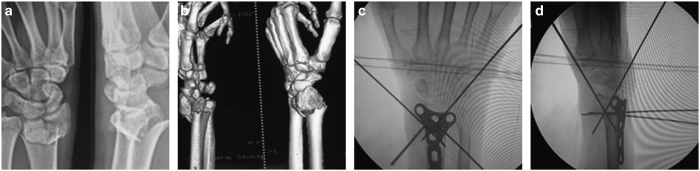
Radiographs of 73-year-old man with a comminuted intra-articular fracture of the distal radius that required treatment via Dorsal locked plating. (a and b) Anteroposterior and lateral radiographs before operation; (c and d) Anteroposterior and lateral radiographs during operation.
